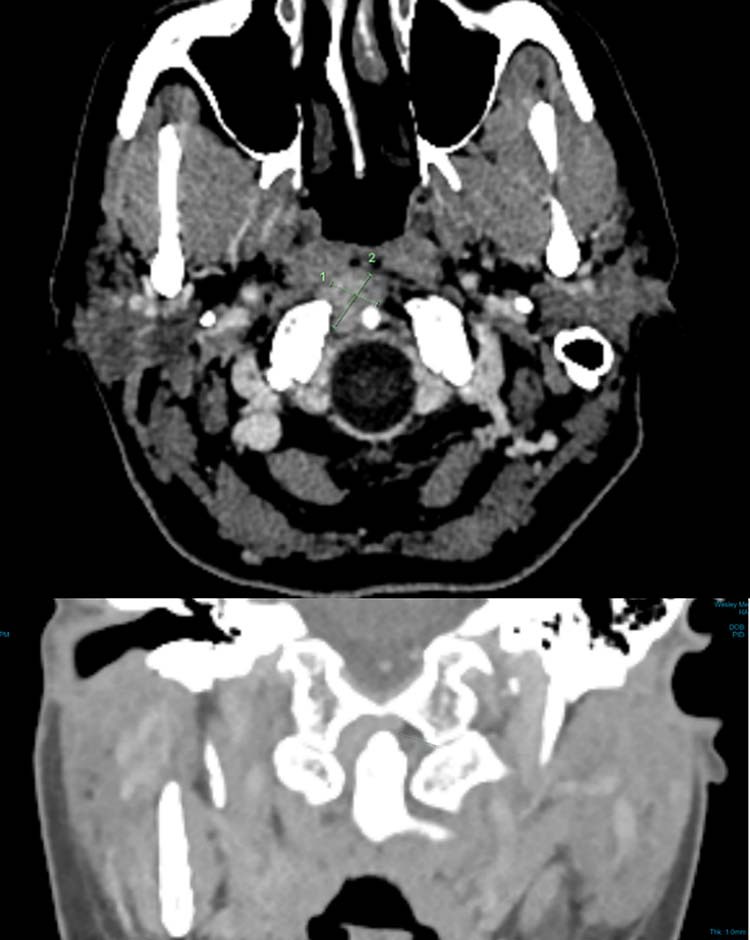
Case 1 CT scan. There was a soft tissue density, measuring 15 x 12 mm (AP x W), superior to the right side of the anterior arch of C1. This extended posteriorly around the right lateral side of the odontoid peg. There was also some minor bony spurring along the superior margin of the anterior C1 articular facet but no bony destruction.
